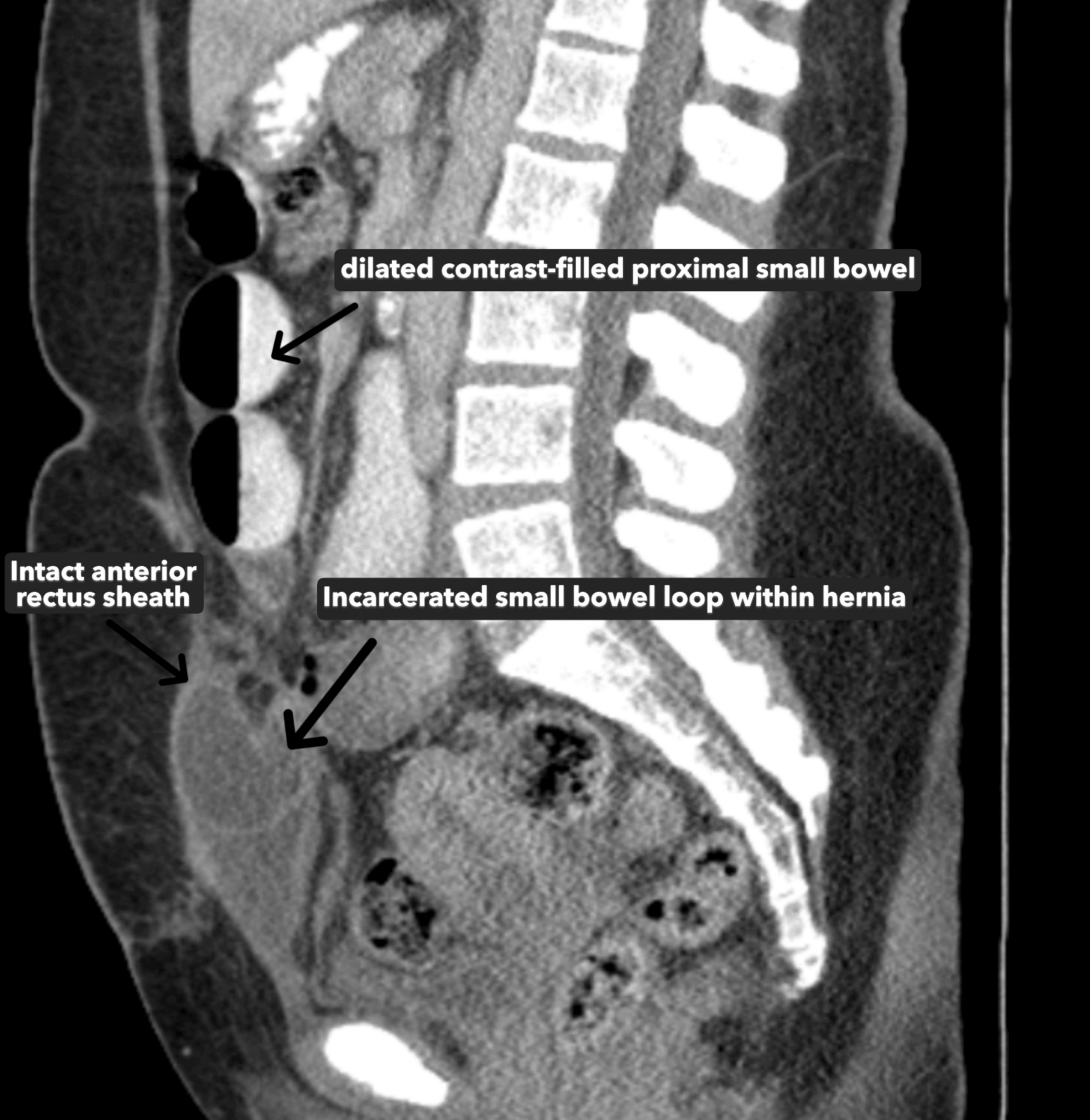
CT scan findings of an incarcerated small bowel loop within the anterior abdominal wall hernia with intact anterior rectus sheath (sagittal view) This sagittal slide of the CT with oral contrast shows a dilated, contrast-filled portion of the small bowel, which is proximal to the site of the hernia. The proximal dilation indicates obstruction of the small bowel at the site of the hernia. The lack of oral contrast within the incarcerated loop is indicative of obstruction at the site of the hernia. This image also shows an incarcerated loop of small bowel within the hernia, with an intact anterior rectus sheath evident. The finding of small bowel between the posterior and anterior rectus sheaths is indicative of an intraparietal hernia.
